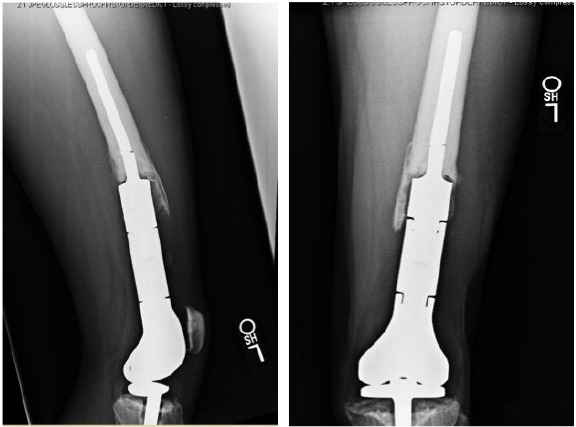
Lateral and anteroposterior radiographs of a broken stem.
